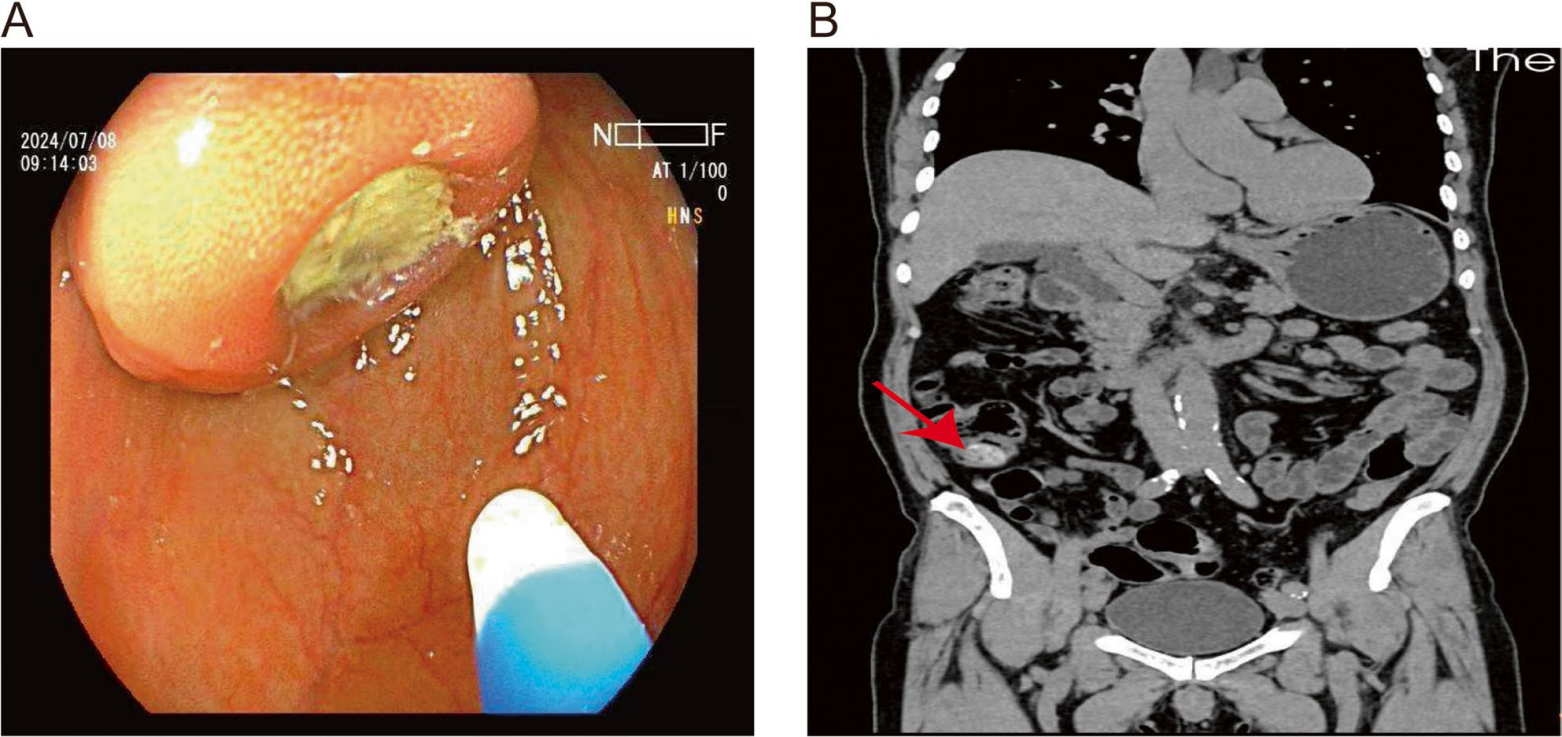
Endoscopic and radiologic features of the inverted colonic diverticulum. A Colonoscopic image of a large sessile cecal lesion with a central depression covered by adherent debris/impacted fecal material. B Coronal contrast-enhanced CT image showing focal thickening of the cecal wall (arrow) with homogeneous enhancement and no malignant features
